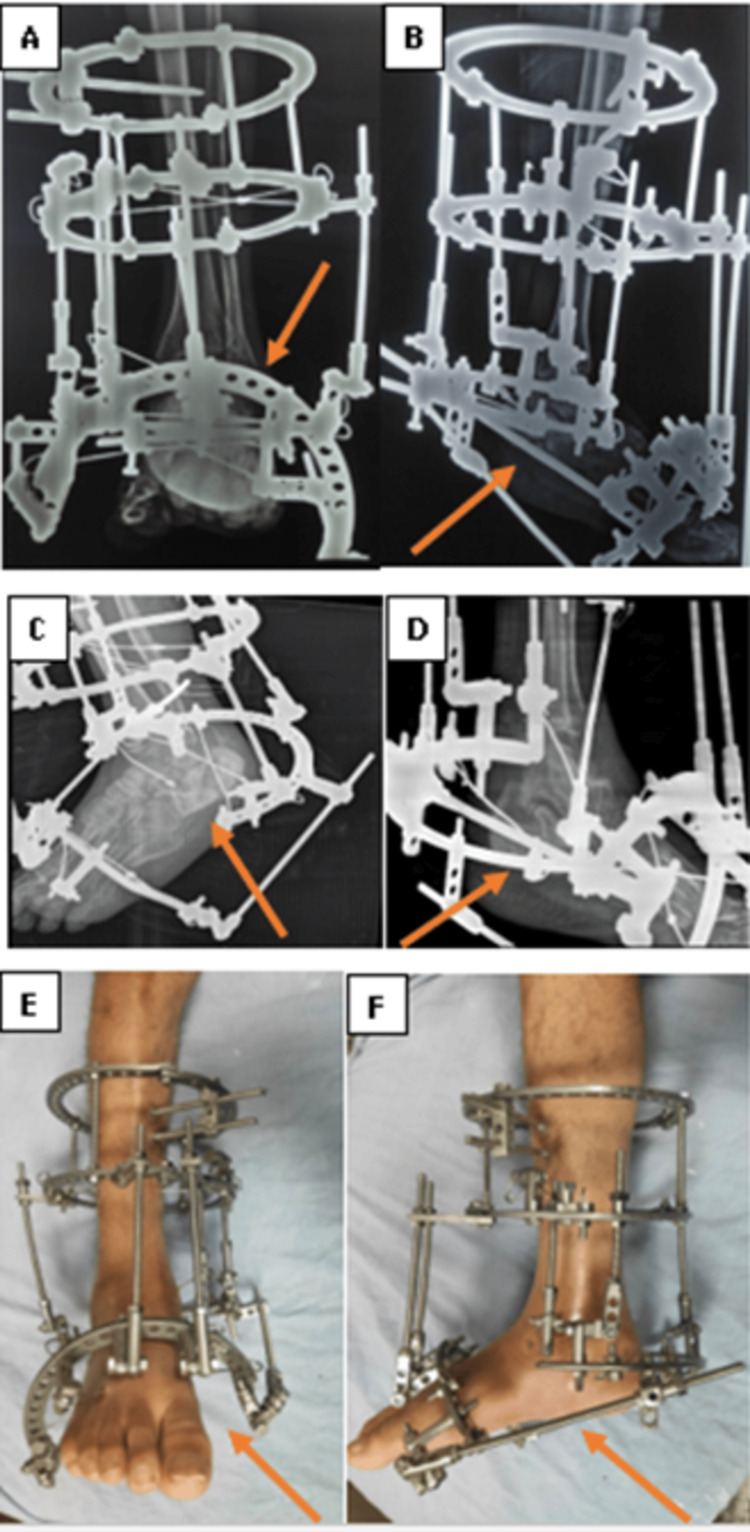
(A, B, C, D) Immediately postoperative plain X-ray. (E, F) Clinical image of the patient frame
